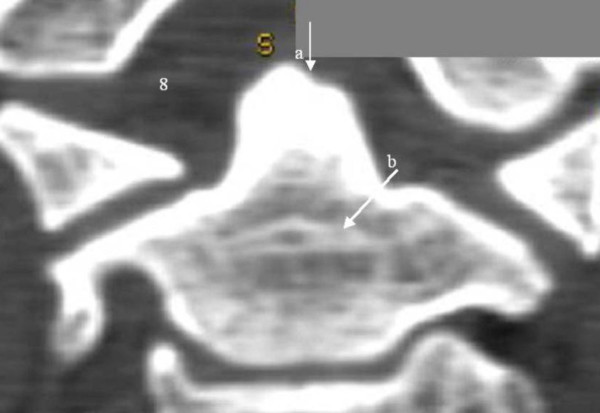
Coronal reconstruction CT scan showed bifid odontoid (arrow1) and subdental synchondrosis (arrow 2).
